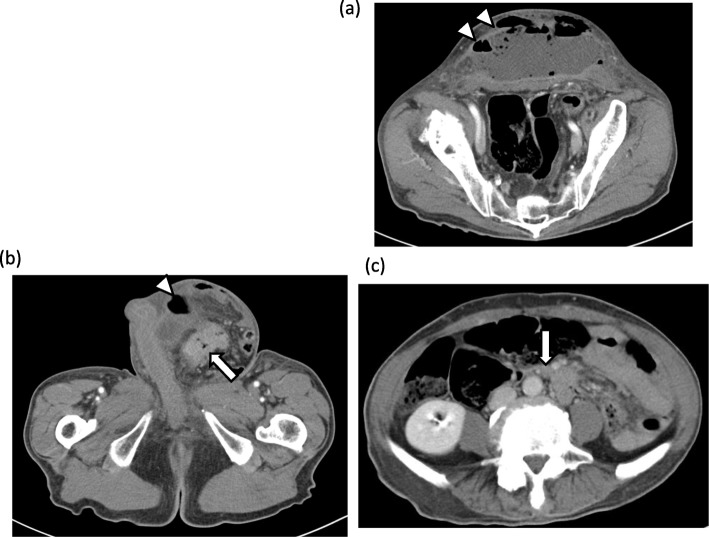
a, b Computed tomography scan showing air-containing fluid in the lower abdominal wall (arrow head), localized wall thickness of the sigmoid colon in the left groin (arrow), and (c) swollen lymph nodes along the IMA and abdominal aorta (arrow)
